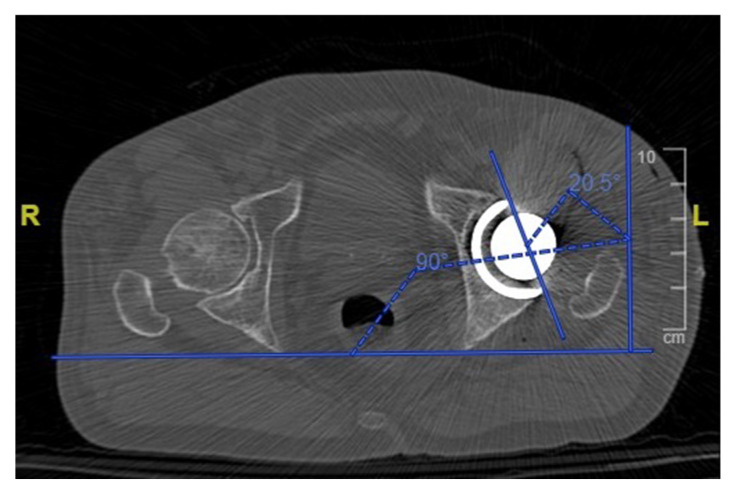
Measurement of the acetabular cup anteversion angle on a postoperative CT scan.
